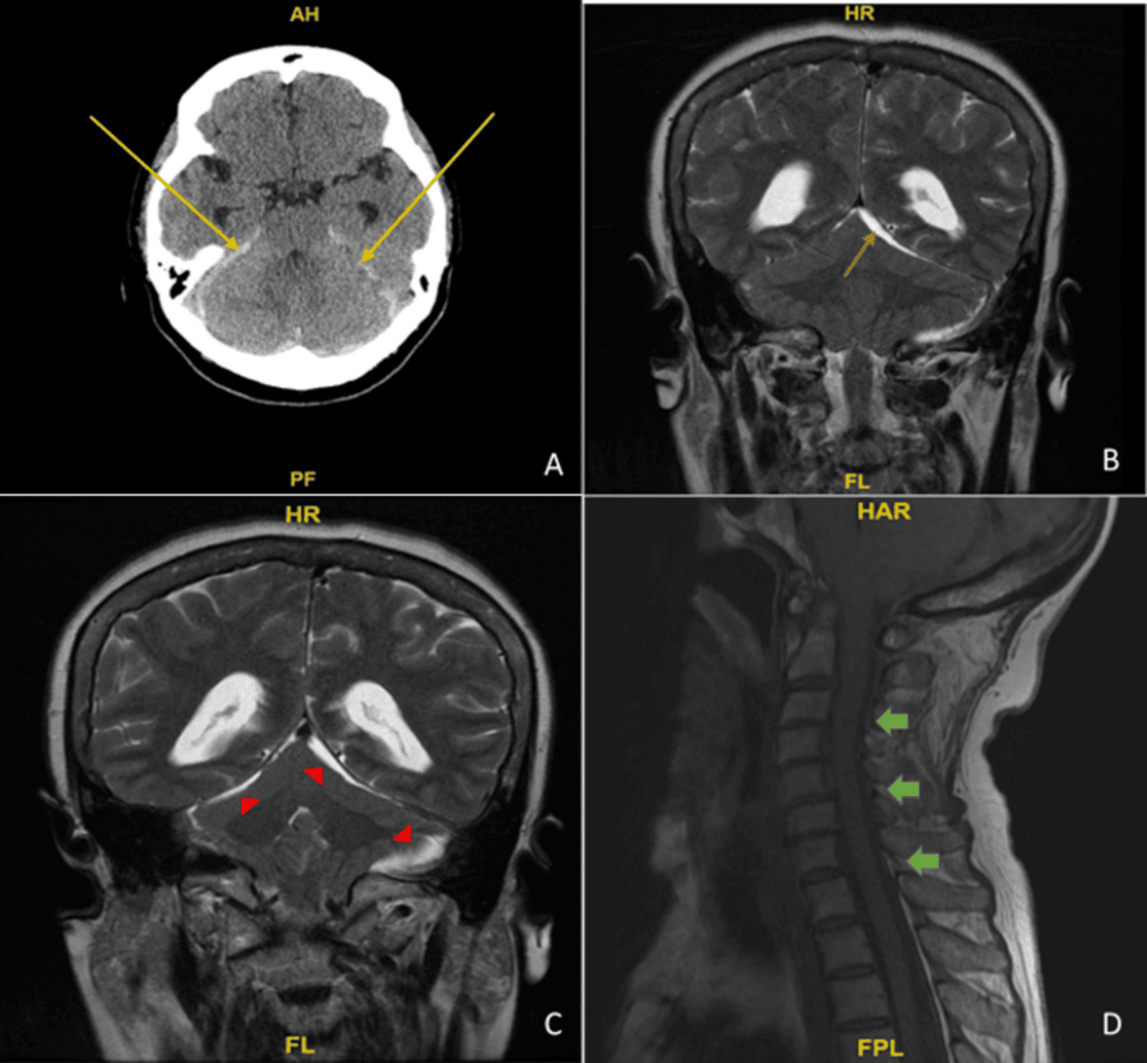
Computed tomography (CT), brain magnetic resonance image (MRI), and cervical spine MRI on admission A: brain CT shows acute subdural blood in the posterior fossa and bilateral tentorium, measuring 5 mm, with crowding of the foramen magnum and suprasellar cisterns as indicated by the yellow arrows; B: T2 coronal brain MRI shows hemorrhagic debris in the tentorium and peripheral aspect of the posterior fossa with inferior displacement or ectopia of the cerebral tonsils highlighted by the orange arrow; C: in the corresponding areas as shown by red arrowheads; D: cervical spine MRI without contrast sagittal T1 demonstrates no significant spinal hemorrhage as demonstrated by green arrows.
